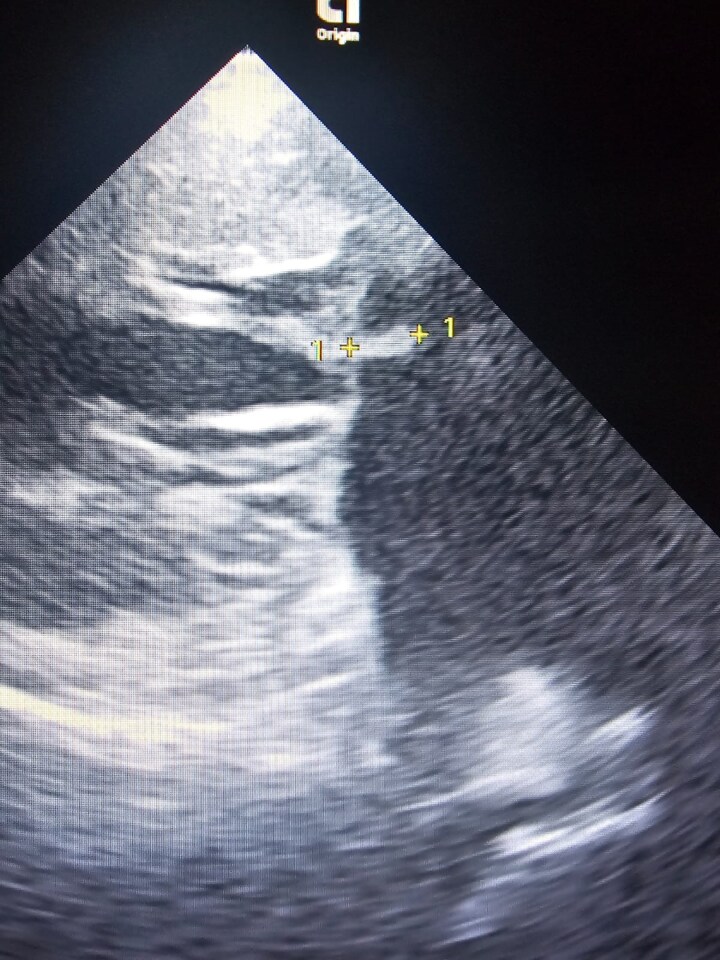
Transthoracic echocardiographic image (parasternal long-axis view): a small echogenic, sessile mass attached to the atrial surface of the anterior mitral leaflet.
